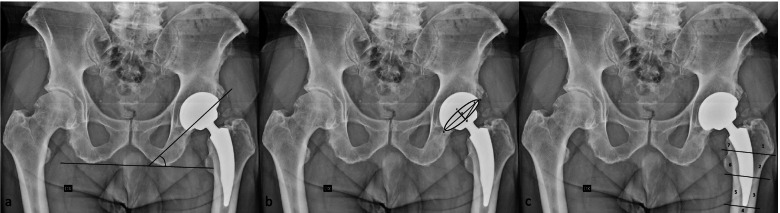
Radiographic evaluation. Techniques of measurement of the inclination acetabular cup (a), ante-version acetabular cup following method explained by Bachal et al. [37] (b) and osteolysis areas according to the Gruen zones (c)
